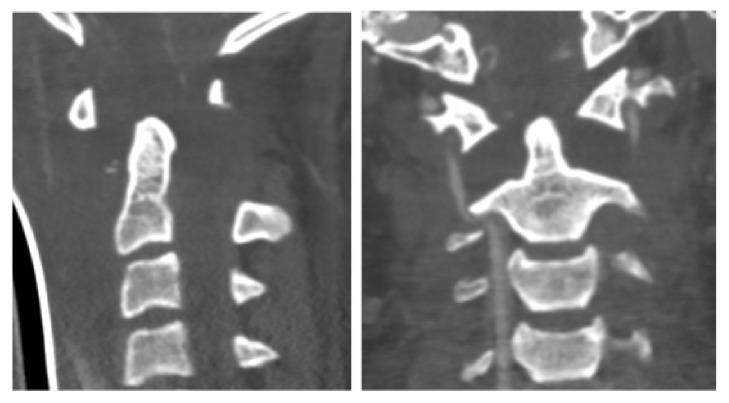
Sagittal and coronal CT reconstruction of C0 to C4, showing traumatic excessive atlantoaxial dissociation.
